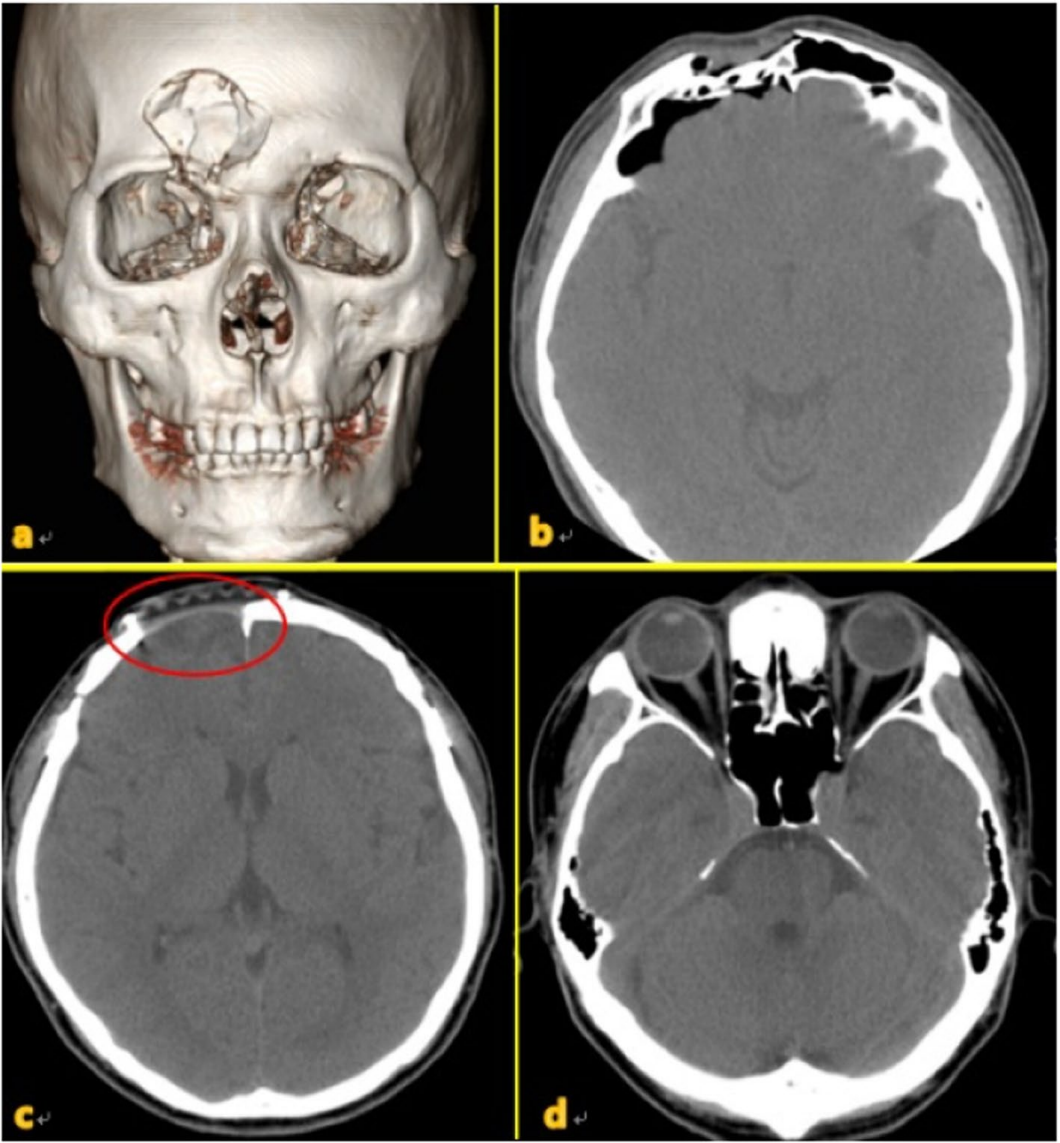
a and b Through-and-through fracture caused by a hammer impact. Pneumocephalus can be observed on the image. c and d CT image acquired at 1 year postoperatively shows anterior expansion of the frontal lobe (red circle). CT, computed tomography
